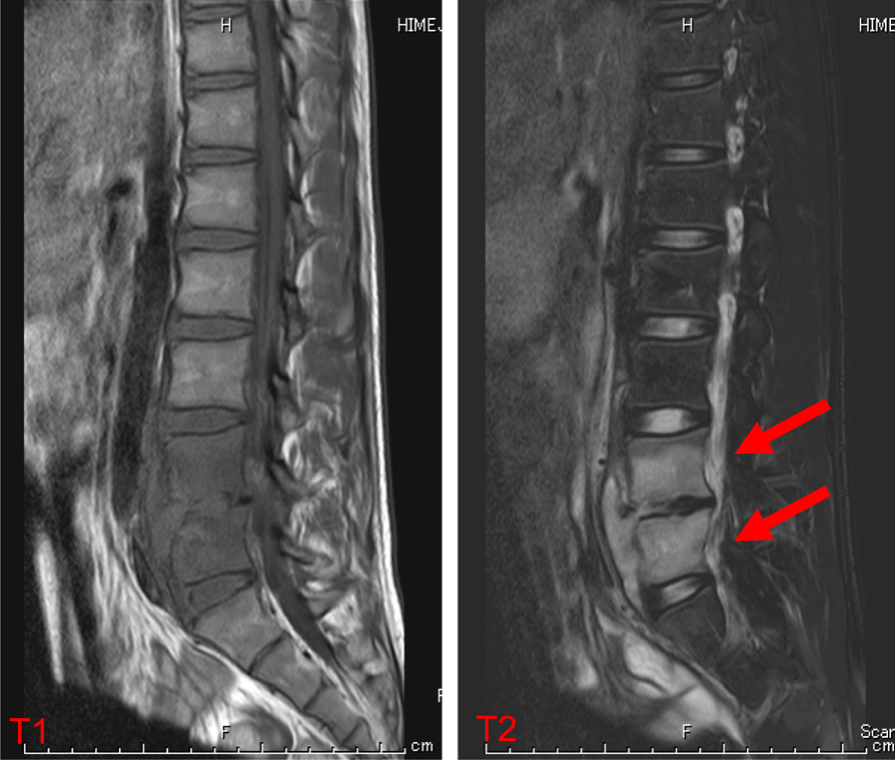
MRI on the day of re-hospitalization showed exacerbation of vertebral disc destruction. Abnormal hyperintensity of vertebral bodies of L4 and L5, and paravertebral soft tissue low diffusion are also seemingly worse than Fig. 2
